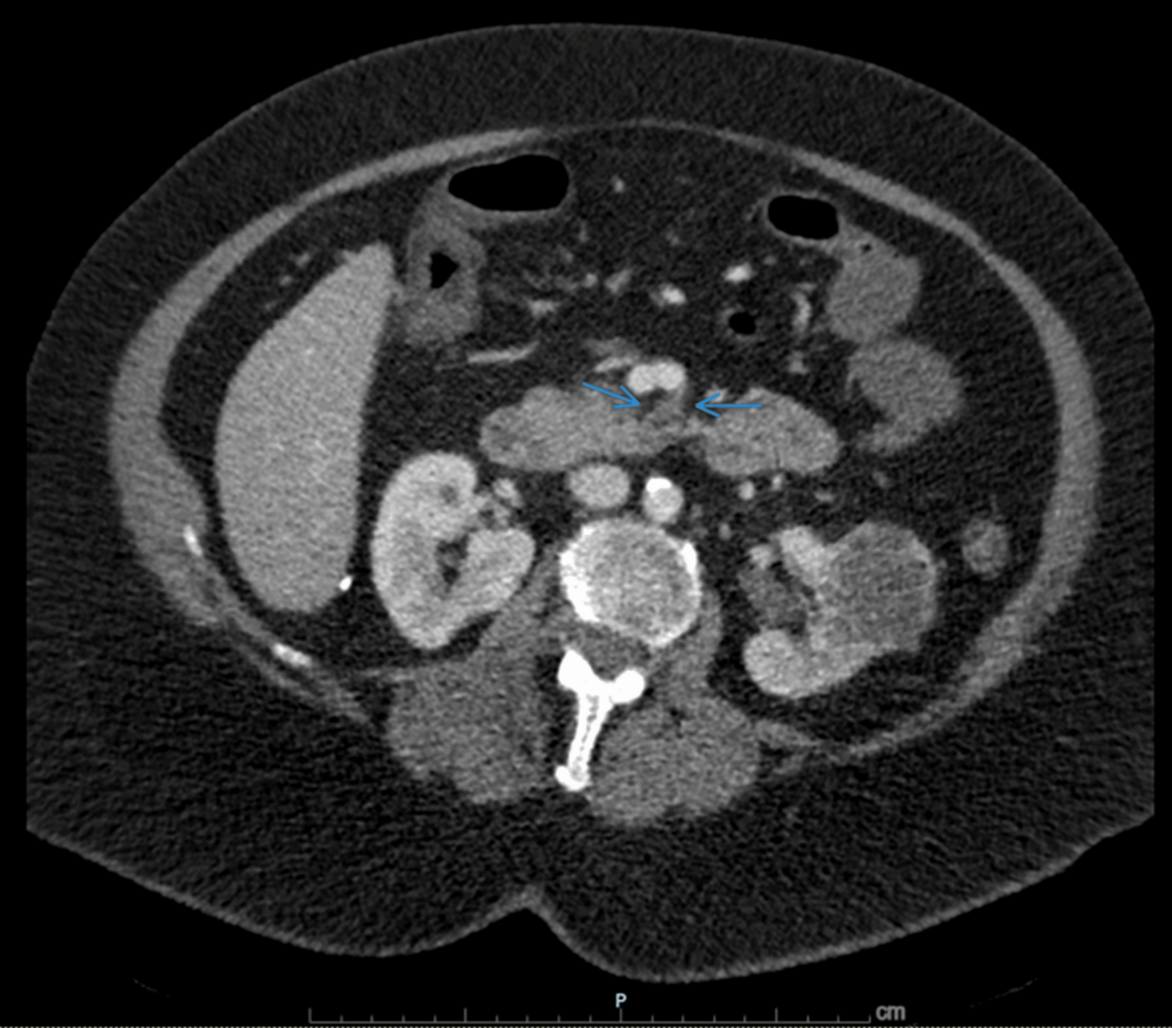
CT angiography demonstrating superior mesenteric artery occlusion. Contrast-enhanced CT of the abdomen demonstrating abrupt nonopacification of the superior mesenteric artery (arrows), consistent with acute arterial occlusion. At the time of imaging, no definite CT evidence of bowel ischemia was present.
